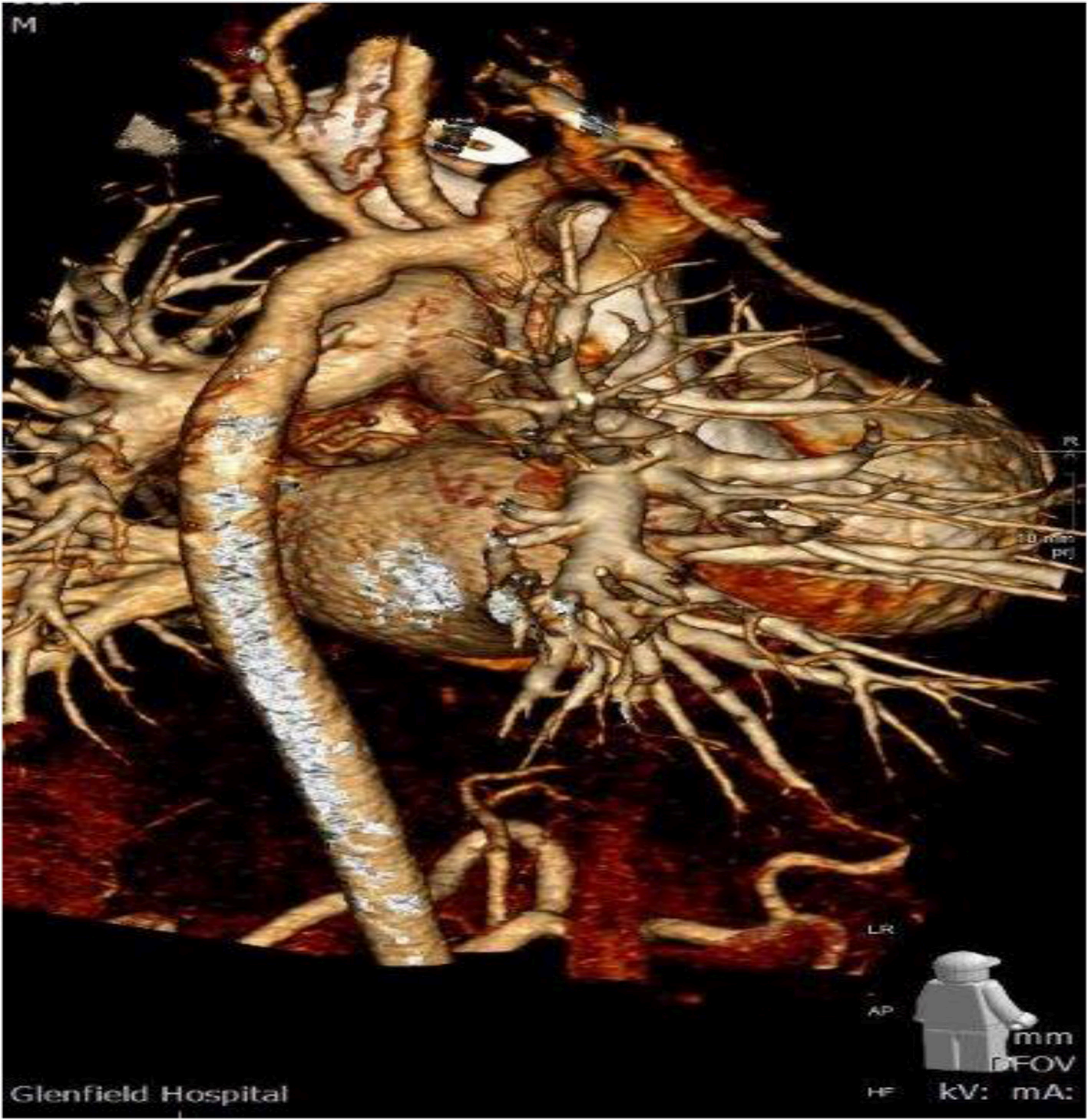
CT scan with 3D reconstruction showing the hypoplastic aortic arch two and half year after DPSIS patch arch reconstruction.
